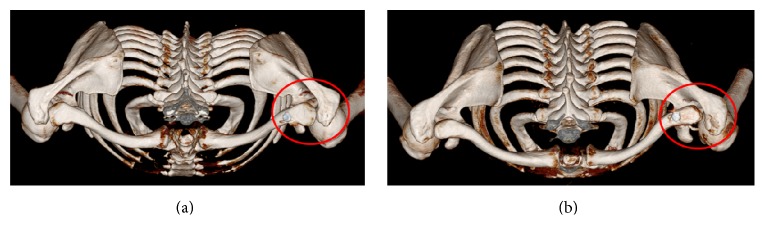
Relationship between distal clavicle and medial acromion with top view of 3D CT. (a) Location of distal clavicle with respect to medial acromion was almost identical to that of contralateral side of distal clavicle, indicating high quality reduction with less than 2 mm difference. (b) Distal clavicle was positioned posteriorly from corresponding articulation of medial acromion, indicating low quality reduction.
